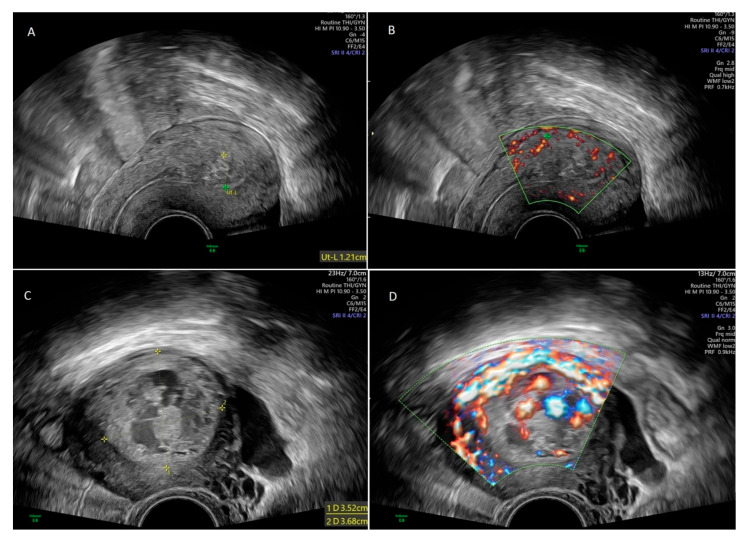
Transvaginal ultrasonography in 30-year old female with retained products of conception (RPOC) (A). Color Doppler ultrasound in the same patient showed minimal vascularity of the lesion (B). Transvaginal ultrasonography in 31-year old female with RPOC (C). Color Doppler ultrasound in the same patient showed hypervascularity of the lesion (D).
